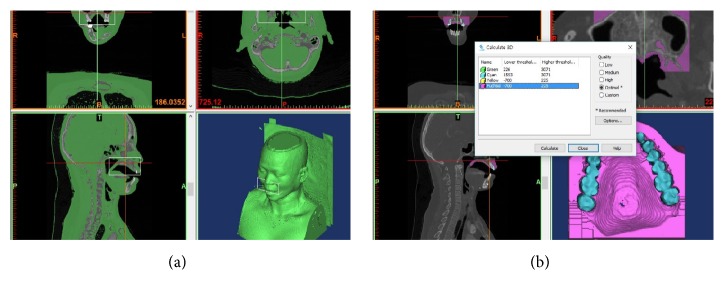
Two-dimensional cross-sectional CT images were stacked, reconstructed 3D model, and segmented palatal mucosa and teeth model. (a) Reconstructed 3D model. (b) Palatal mucosa and teeth model.
